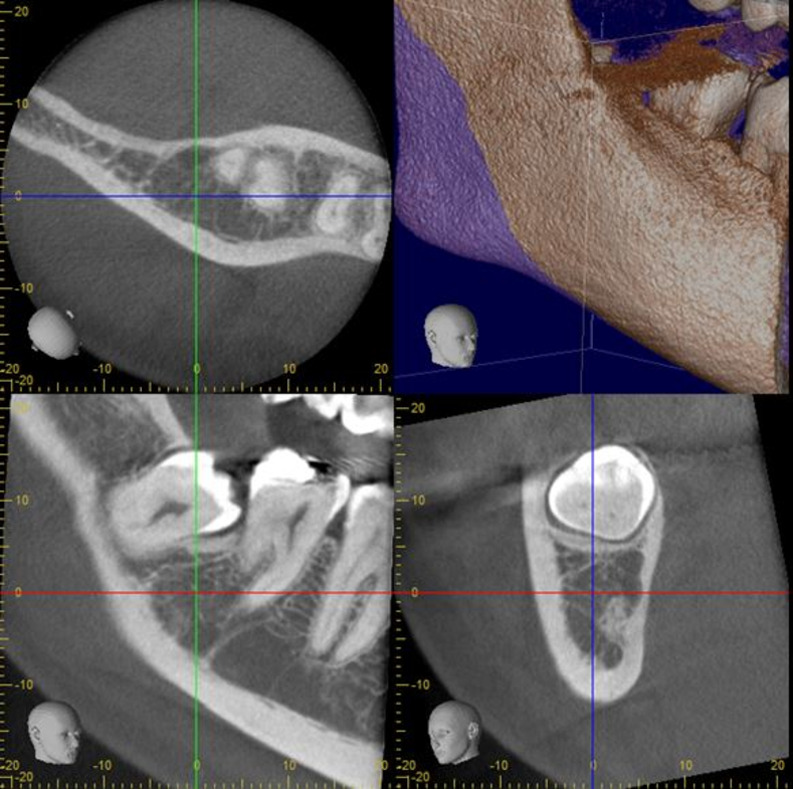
An image of a patient’s CBCT planes.
